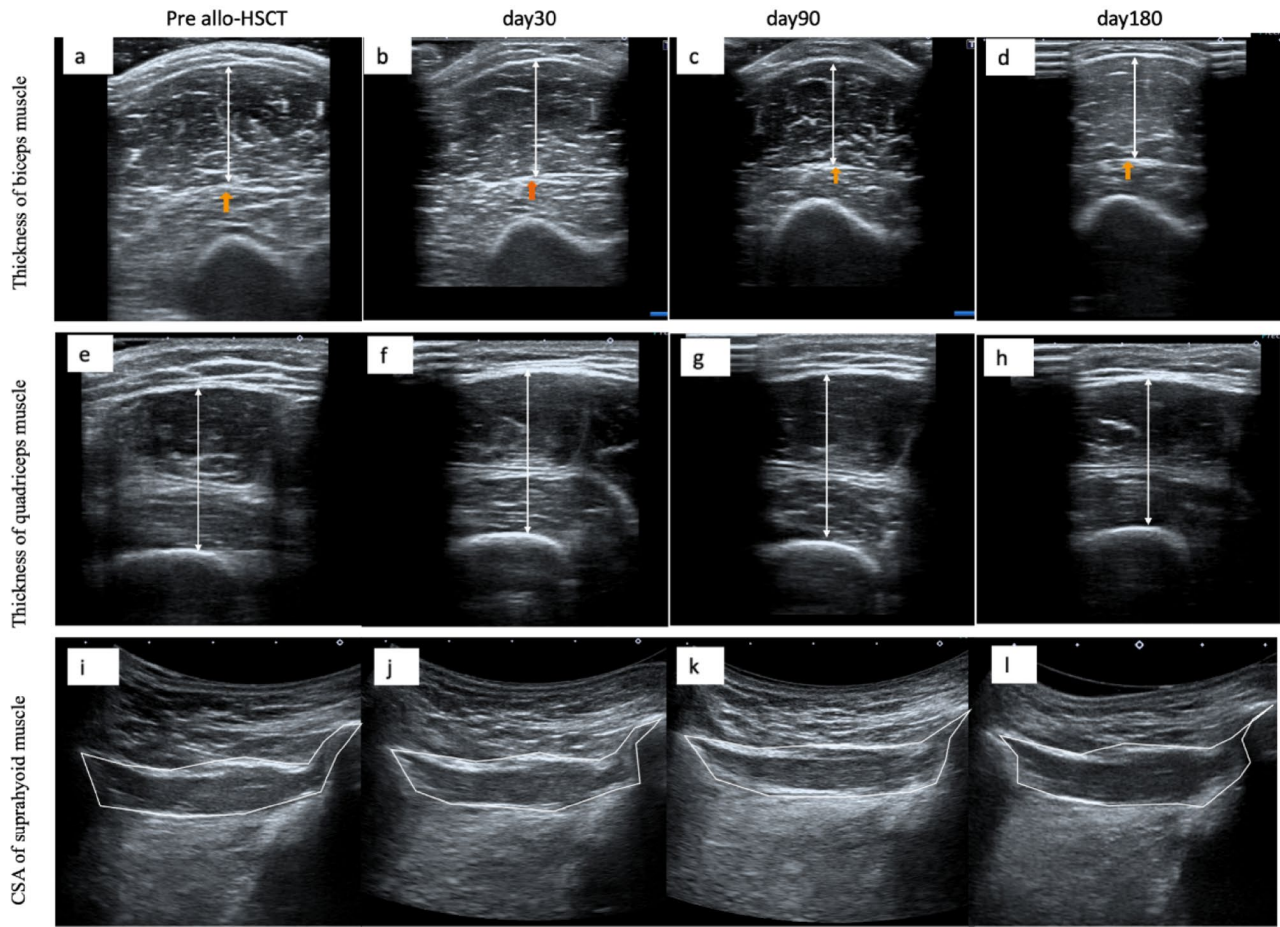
Ultrasound images of the biceps muscle (a–d), the vastus intermedius muscle and rectus femoris muscle complex (e–h), and the geniohyoid-mylohyoid muscles complex (i–l) before and on days 30, 90 and 180 after allo-HSCT, respectively.
